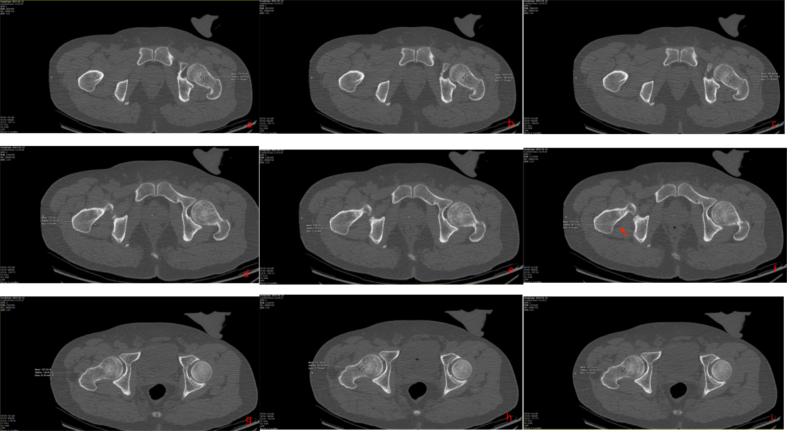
Comparative CT attenuation measurements are presented.
